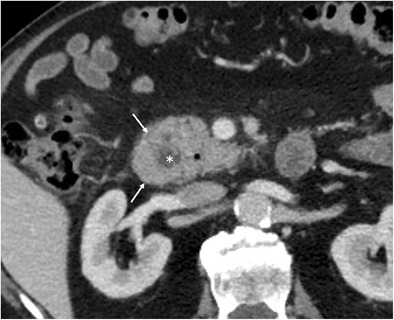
Annular pancreas in a 43-year-old man with a long history of abdominal pain. Contrast-enhanced CT shows the pancreas (arrows) completely encircling the descending duodenum (*)
